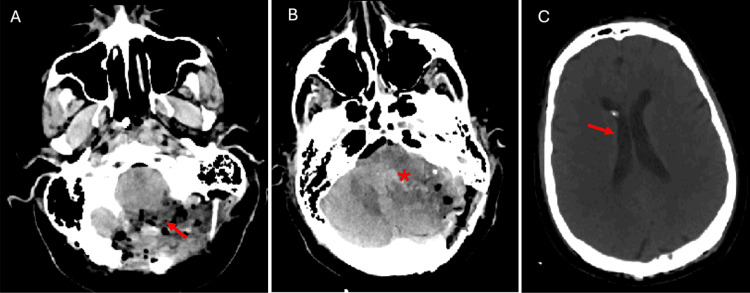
The patient's computed tomography of head (CTH) post suboccipital craniectomy and C1 laminectomy Non-contrast axial CTH demonstrating postsurgical sequela from suboccipital craniectomy and removal of old titanium mesh with C1 laminectomy. A small volume of subcutaneous gas overlying the operative site with extension to the posterior neck is demonstrated (panel A, red arrow). A similar degree of vasogenic edema is appreciated with continued effacement of fourth ventricle and posterior fossa cisterns (panel C, red asterisk). There is a significant decrease in size of the lateral ventricles with frontal approach external ventricular drain (EVD; panel C, red arrow).
